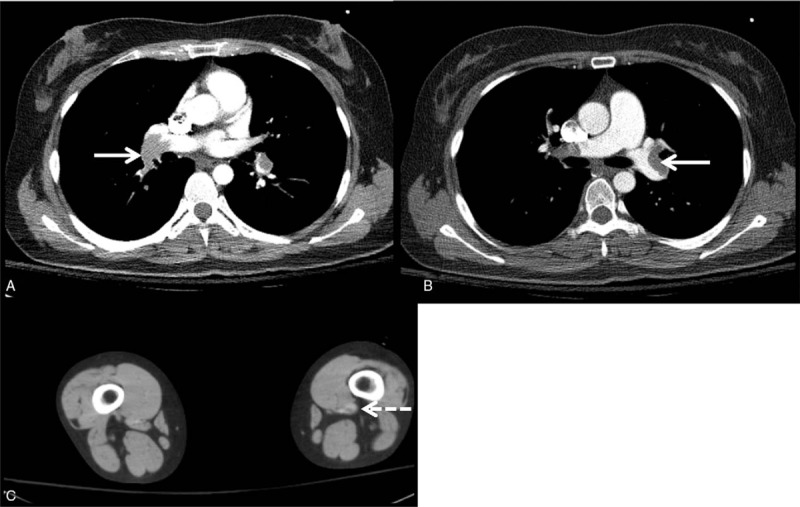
Contrast-enhanced computed tomography at initial visit. (A, B) Thromboembolism in both main pulmonary arteries and upper, middle, and lower lobar pulmonary arteries (*arrow* indicates the right and left pulmonary arteries, respectively) (C) Deep vein thrombosis in the left popliteal vein (*dotted arrow*).
